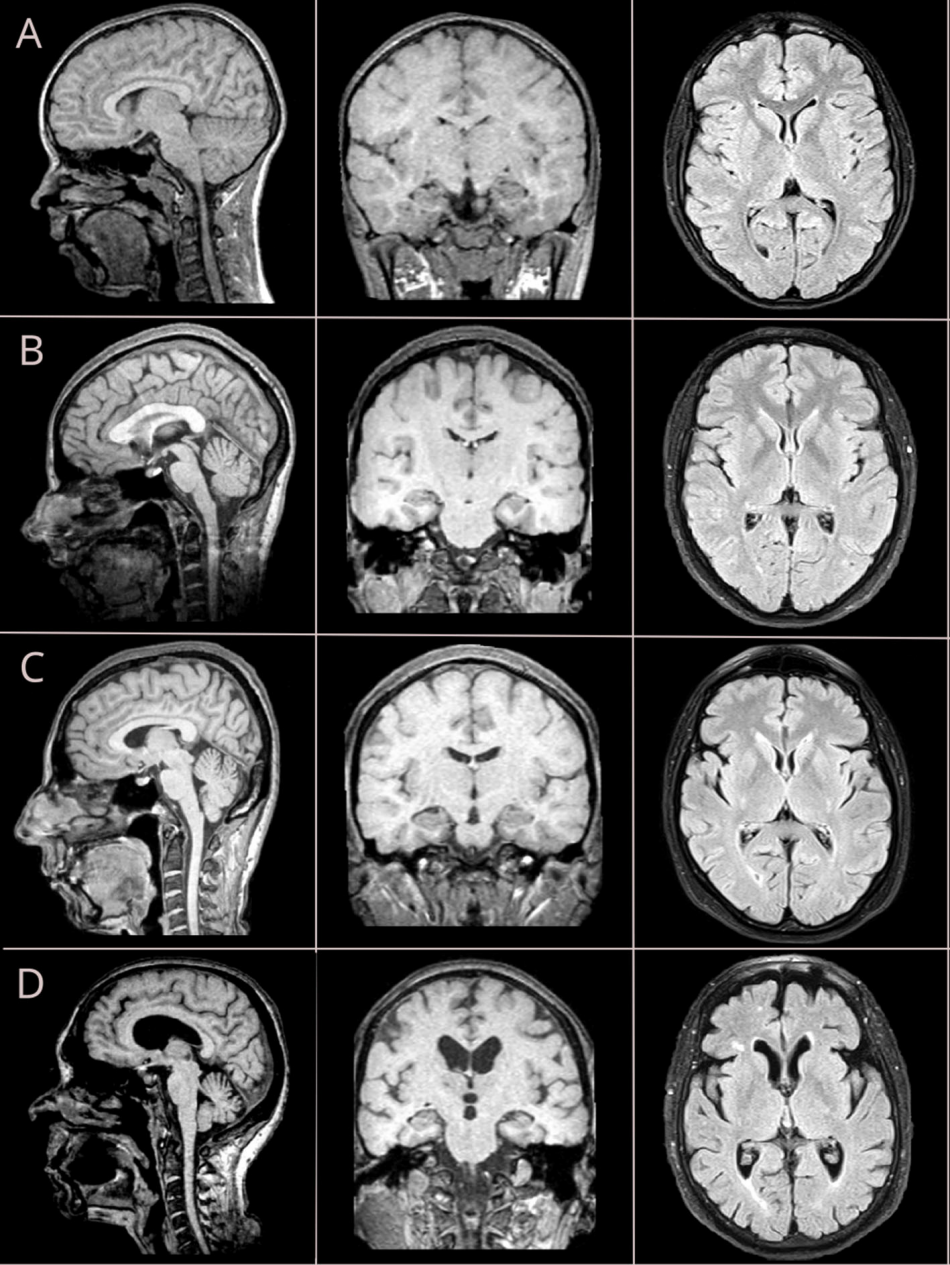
Brain MRI changes across four life periods: adolescent age (A), young adulthood (B), midlife age (C), and older adulthood (D). Sagittal and coronal views are reconstructed from T1-weighted (T1W) sequence with isometric (3D) vowel; axial views are retrieved from fluid-attenuated inversion recovery (FLAIR) sequence.
